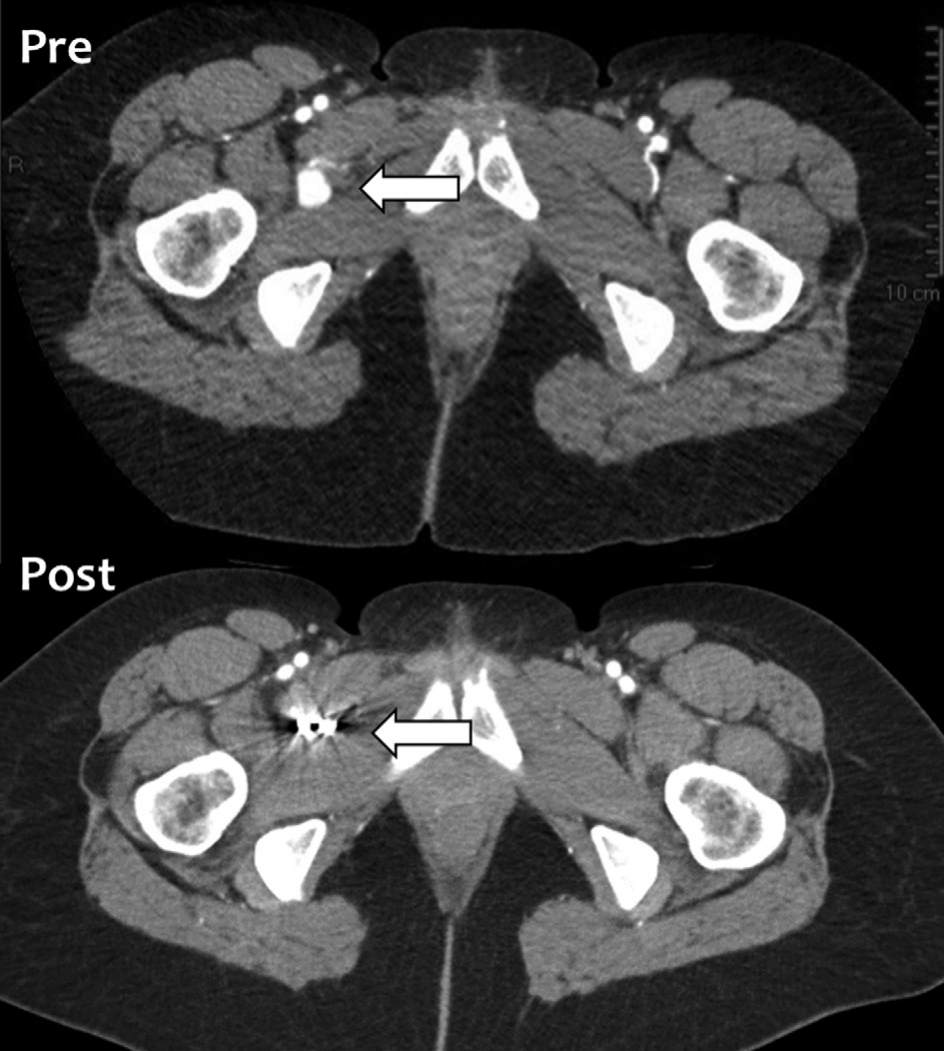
A CT appearance of pre- and post-embolisation. A pseudoaneurysm was embolised with micro coils (arrow).
